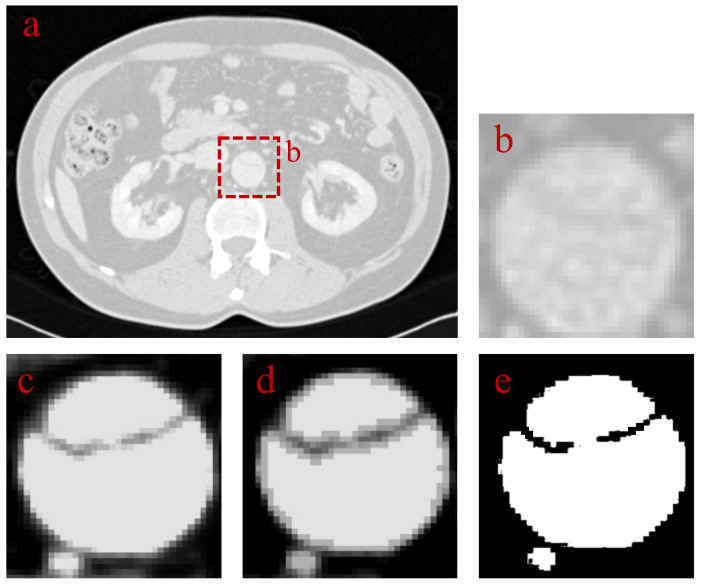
Image-based procedure for reconstructing the aortic geometric models: (a) example of DICOM image from the FPA CT scan sequence; (b) identification of the region of interest; (c) enhancement of image contrast; (d) smoothing of lumen boundaries through the ad hoc developed image filter; and (e) image binarization.
